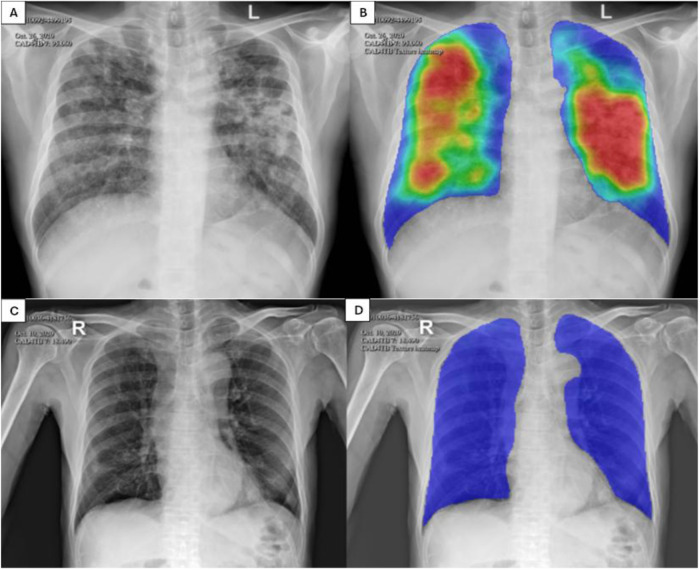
(A) example of original chest x-ray image of a positive tuberculosis patient confirmed by AFB smear. (B) The output of CAD4TB analysis from (A) with a score of 95.06. (C) Example of original chest x-ray image of a negative tuberculosis patient confirmed by AFB smear. (D) The output of CAD4TB analysis from (C) with a score of 18.49.
